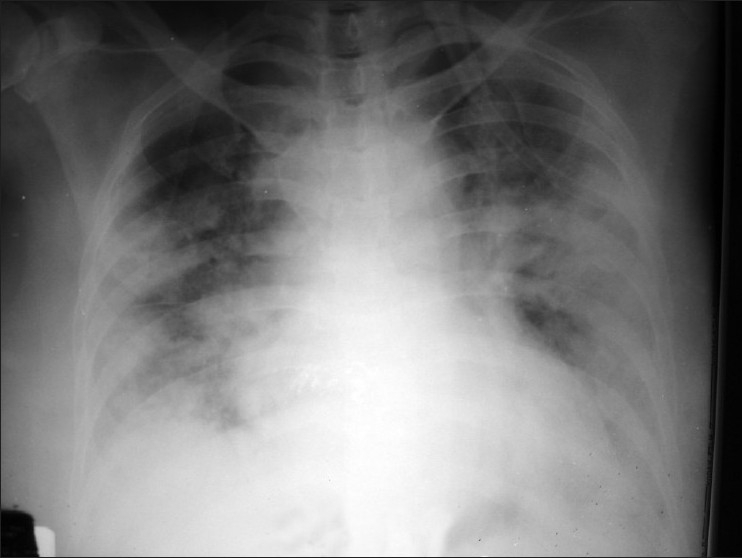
Chest radiograph showing ill-defined bilateral air-space opacities
